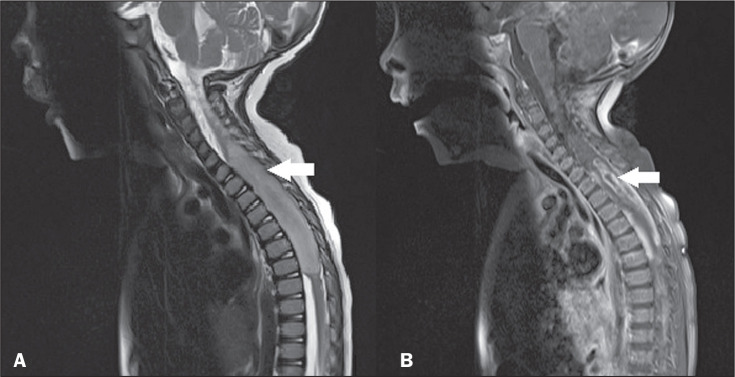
Astrocytoma. Sagittal MRI scans. A: T2-weighted sequence showing a spinal
